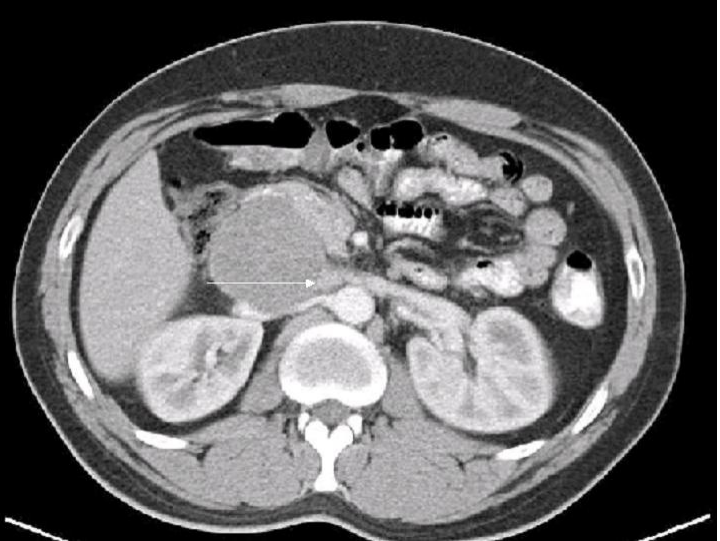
Abdominal CT-scan. Axial contrast enhanced CT image showing a retroperitoneal tumor with obstruction of the IVC and involvement of the left renal vein (arrow).
